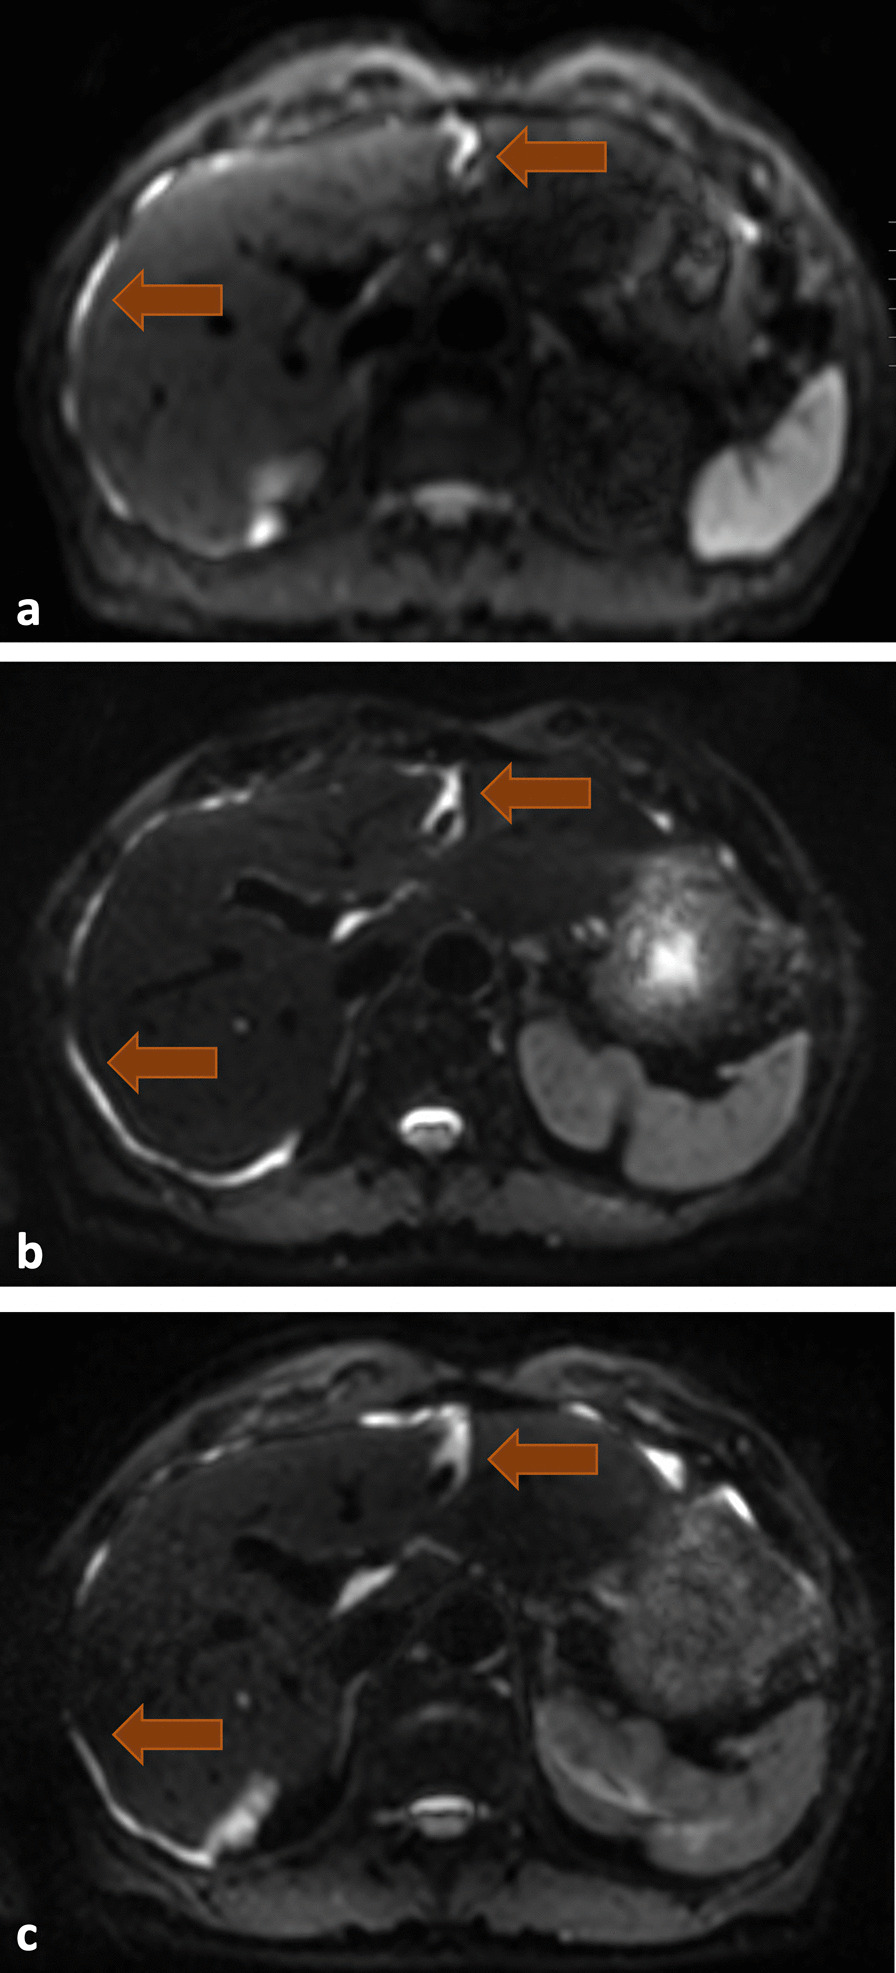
MRI scan with diffusion-weighted imaging (DWI) sequence shows mucinous implants as bright spots around the liver and in the falciforme ligament (red arrows). Subfigure a shows initial findings, b 1 year afterwards and c shows the stable findings 4 years after initial diagnosis
